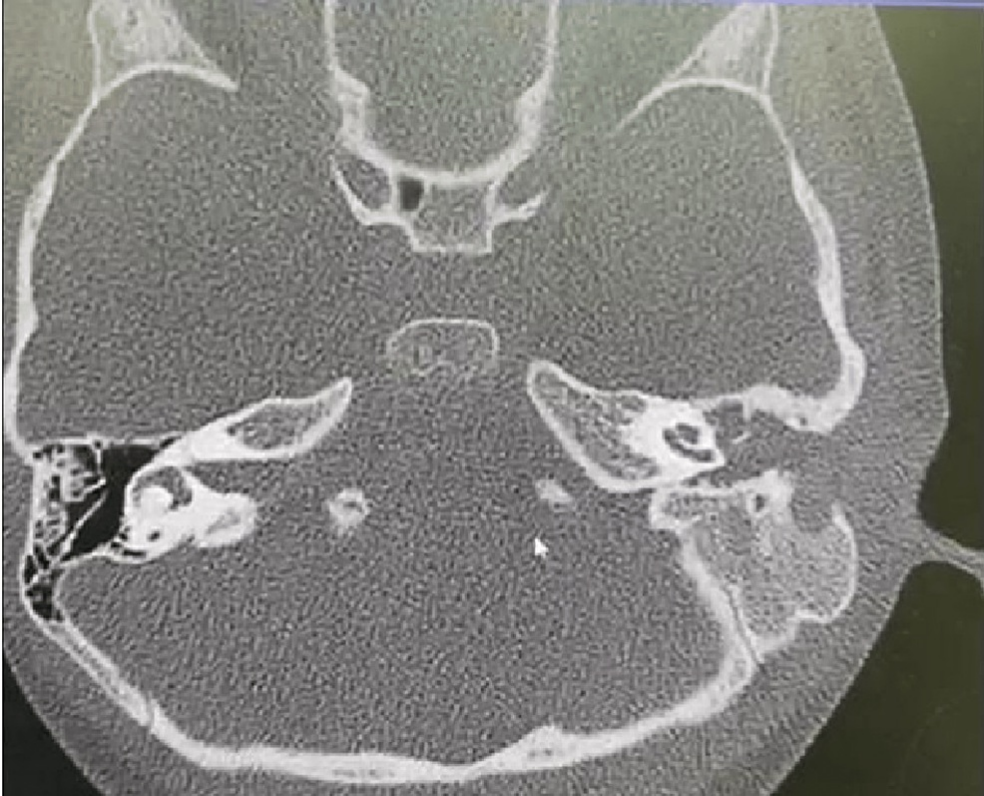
Non-enhanced temporal bone computed tomography scan showing soft tissue lesion totally obliterating the left middle ear cavity and left mastoid air cells, engulfing its bony ossicles
